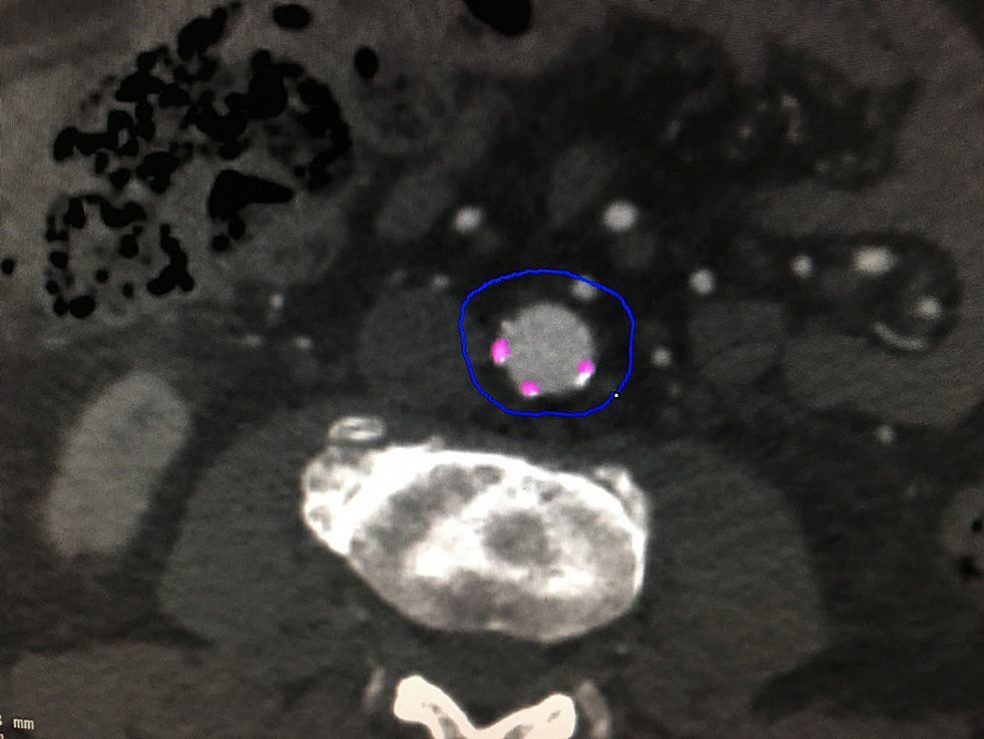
Calcium scoring using Aquarius workstation; TeraRecon software. Axial CT slice demonstrating selection of the plaques (which are highlighted in pink).
